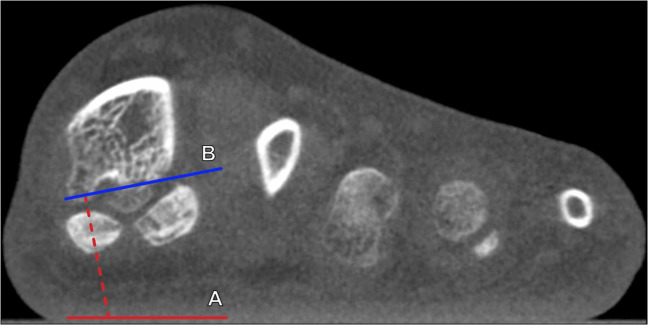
Measurement of the first metatarsal pronation angle on weightbearing CBCT of the foot. Measured on standing sesamoid tangential radiographs or a coronal weightbearing CBCT, the angle is formed between a horizontal line (A, red line) along the weightbearing surface of the foot and a line (B, blue line) from the lateral edge of the lateral hallux sesamoid sulcus to the medial edge of the medial hallux sesamoid sulcus. The first metatarsal pronation angle quantifies the degree of first metatarsal axial rotation along its long axis with lower (more negative) values indicating supination, and greater (more positive) values indicating greater pronation
